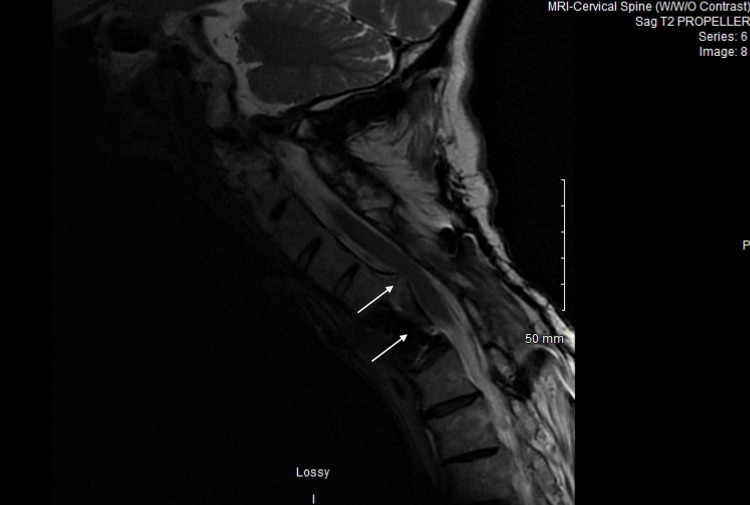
Sagittal T2-weighted MRI of the cervical spine demonstrating severe anterior cervical cord compression at the C4–C6 levels.
